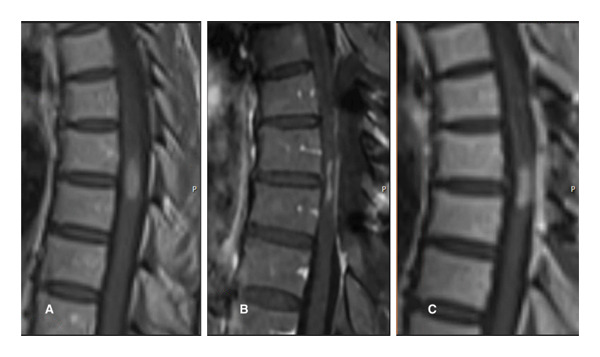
Sagittal T1 postcontrast MRI revealing homogenously enhancing intramedullary lesion at T7–T8 with associated cord edema and rostral syrinx formation immediately before (A) and after (B) T6–T8 laminectomy/laminoplasty for biopsy/subtotal resection. Subsequent MRI at 1‐month postop (C) reveals regrowth of the lesion.
